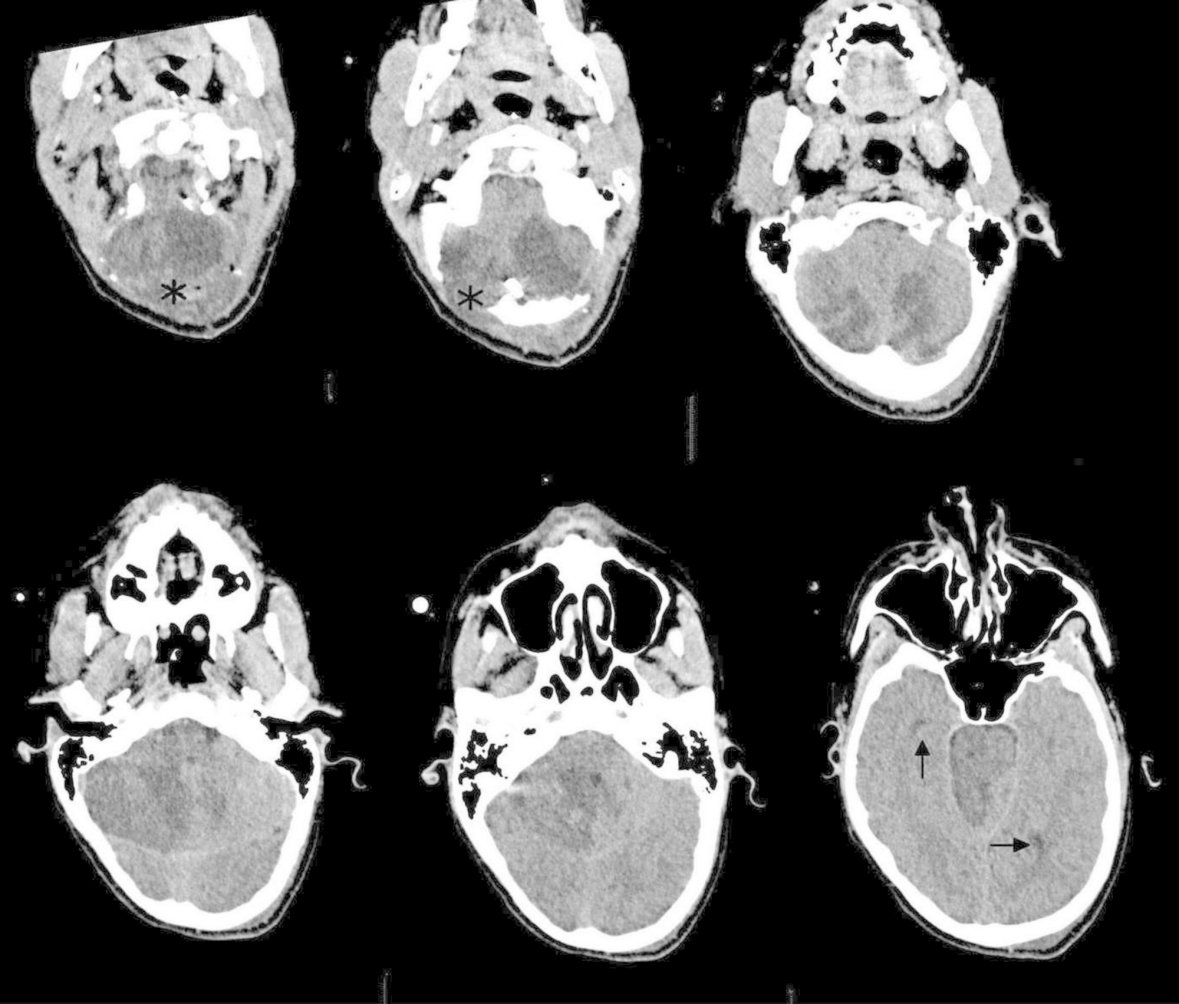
Computed tomography (CT) scan five days after neurosurgical treatment CT scan five days after occipital craniectomy (black stars) demonstrates physiological visualization of the cranial subarachnoid spaces due to the reduction of the compressive effect (black arrows).
